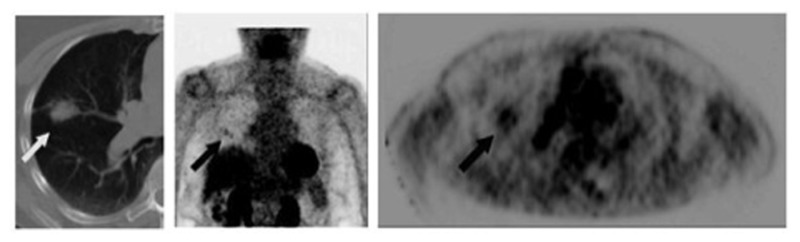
Eighty-two-year-old female with growing right lung nodule. Prior lung CT scans over a 1-year period had demonstrated increasing size of a spiculated-appearing right lung nodule (white arrow). FDG-PET/CT was performed for nodule characterization. Images show corresponding transaxial planes through the chest from the CT and FDG PET (*upper right*); and a 3D maximum intensity projection imaged centered on the chest region (*center image*). PET showed detectable, albeit minimal, activity in the right lung nodule (black arrows; SUV_max_ 2.1) and focal activity in the right pulmonary hilum (arrowhead) thought to represent lymph node FDG uptake. Wedge resection was performed; pathology found focal bronchopneumonia/granuloma.
